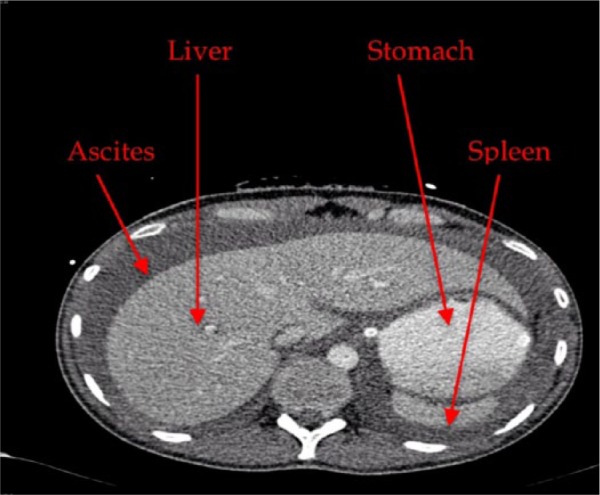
A slide from CT abdomen that shows that the patient is very thin with large ascites and very large liver.
